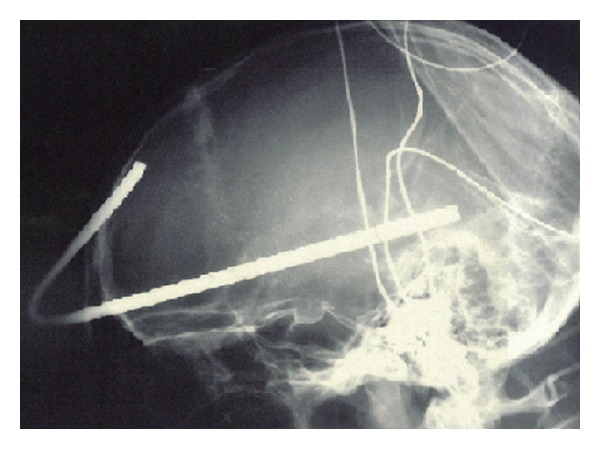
Lateral plain X-ray of the skull demonstrates the trajectory of the metallic rod, which enters approximately 1 cm above the supraorbital rim, involves the frontal sinus, and points through the right temporal lobe for an intracranial length of 14.5 cm.
